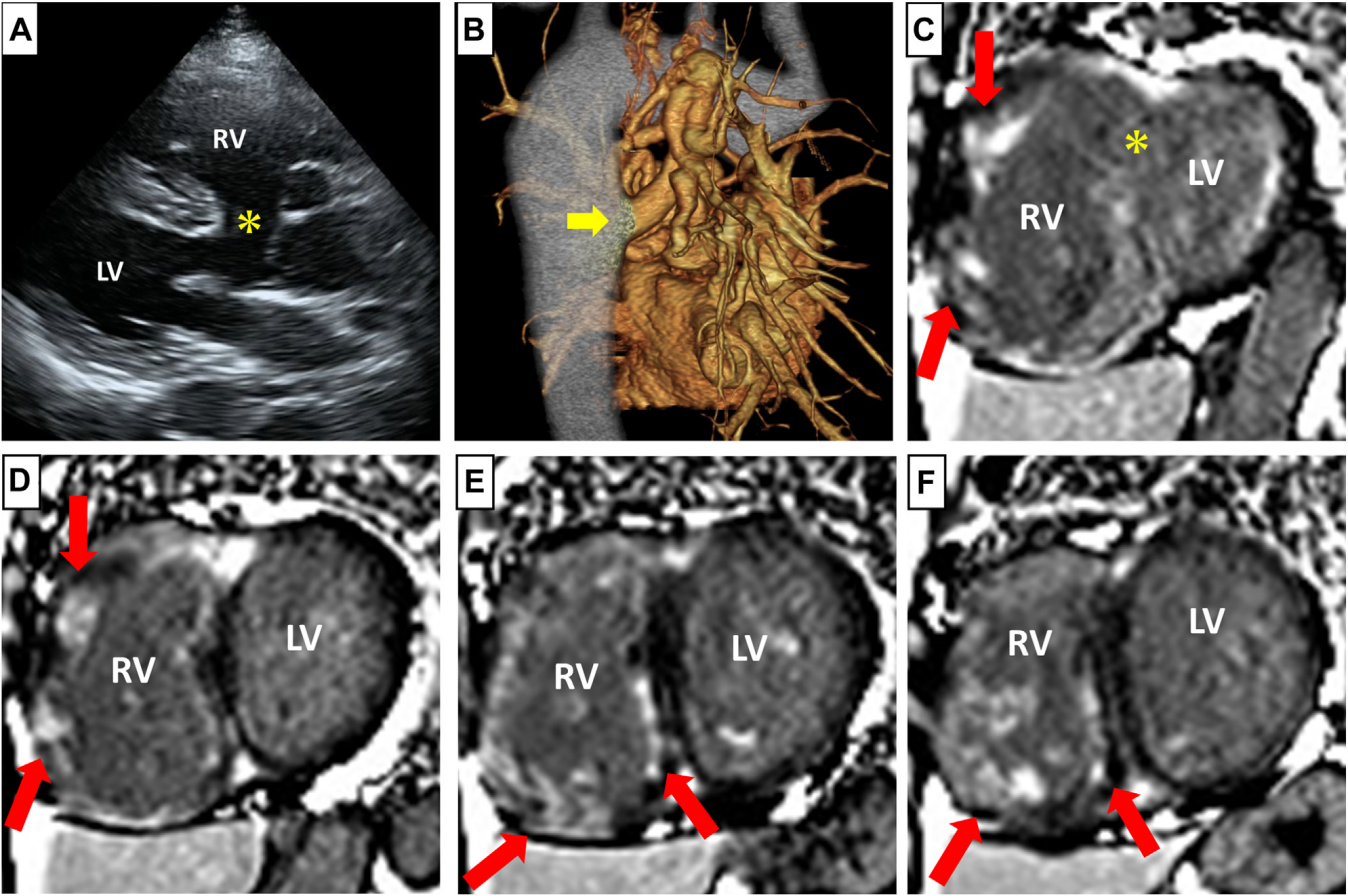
(A) Parasternal long-axis view on transthoracic echocardiography showing the VSD (asterisk), aortic override, and RV enlargement. (B) Right posterior oblique view of the 3-dimensional reconstruction of contrast-enhanced computed tomography. A MAPCA from the descending aorta was observed (arrow). (C-F) Short-axis stack of gadolinium-enhanced cardiac magnetic resonance imaging in alphabetical order from base to apex. At the base, the VSD was observed (asterisk), and late enhancement was prominent in the contralateral RV free wall (arrow). Toward the apex, late enhancement of the septum and papillary muscles on the inferior wall was prominent (arrow). LV, left ventricle; MAPCA, major aortopulmonary collateral artery; RV, right ventricle; VSD, ventricular septal defect.
